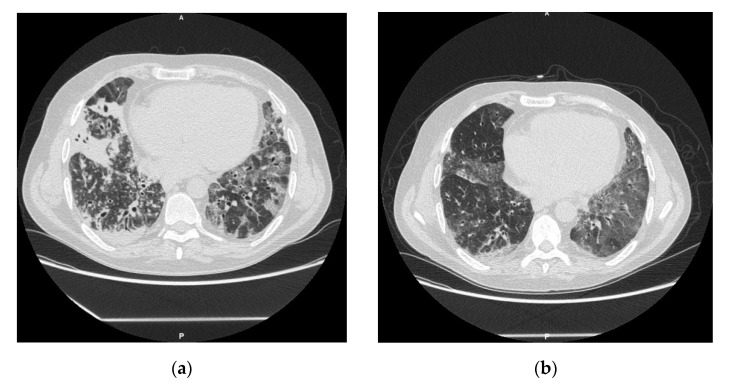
(a) Presentation CT scan of Case 1; (b) CT scan after antifungal treatment.
